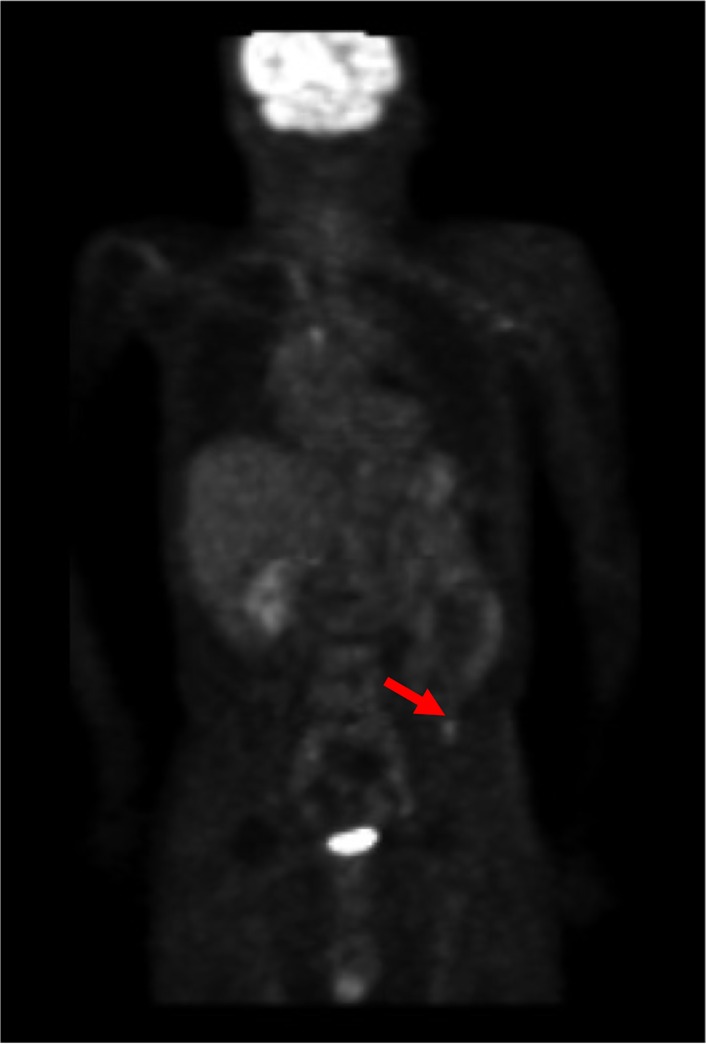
Example of abnormal accumulation (colon cancer). The SUV of 2.1 was converted into Z-score of 5.73, because the mean and SD at the location (an arrow) were 0.61 and 0.26, respectively.
